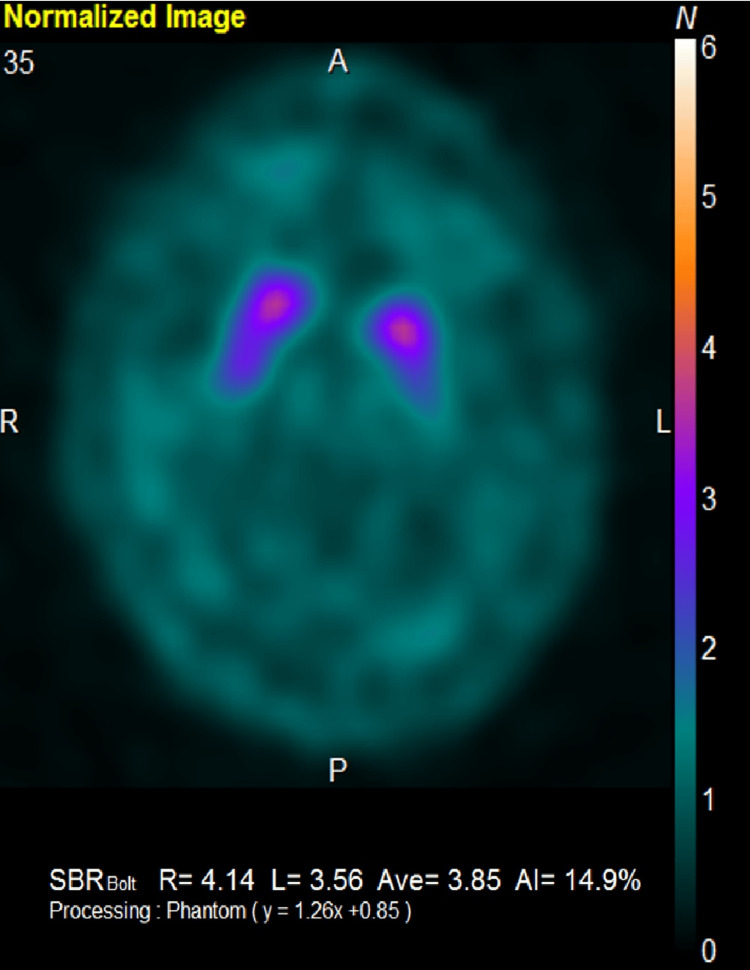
Results of the DAT-SPECT scan The DAT-SPECT scan revealed an asymmetric reduction in radiotracer uptake within the striatum. The specific binding ratio was 4.14 on the right and 3.16 on the left, respectively. DAT-SPECT: Dopamine transporter single-photon emission computed tomography
